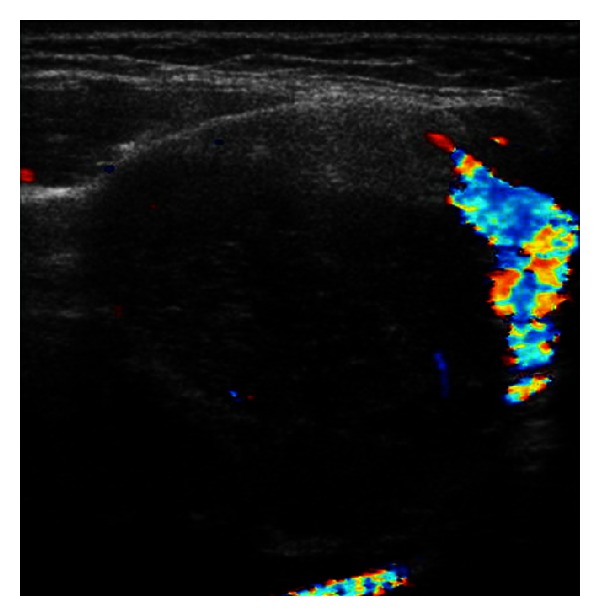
Ultrasound right neck mass.
